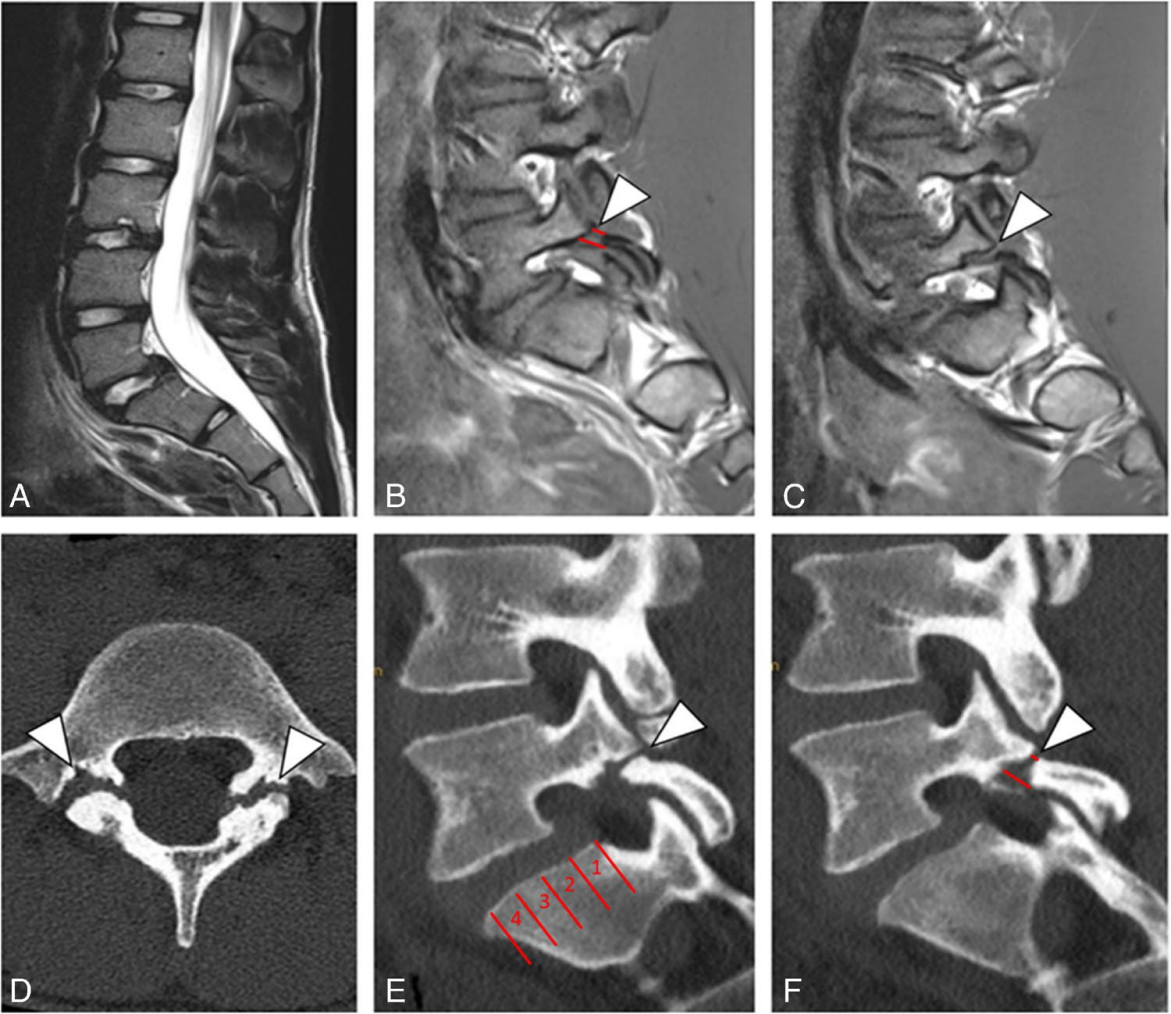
A Baseline sagittal T2-weighted MRI image of a 17-year-old male shows anterolisthesis Meyerding grade I at the level L5/S1 with an anterolisthesis distance of 6 mm. B T1-weighted sagittal MR image on the left side with lysis gap (arrowhead). Red lines indicate the measurement of anterior and posterior lysis gap at the level of the facet joints. C T1-weighted sagittal MR image on the right side with lysis gap (arrowhead). D Axial follow-up CT image of the same patient (now 24 years old) with persisting bilateral lysis (arrowheads). E Sagittal follow-up CT image on the left side with persisting lysis gap (arrowhead). The severity of spondylolisthesis was graded according to the Meyerding classification, dividing the slippage in relation to the quarter of the superior endplate of the vertebra below, and F sagittal follow-up CT image on the right side with lysis gap (arrowhead). Red lines indicate the measurement of anterior and posterior lysis gap
